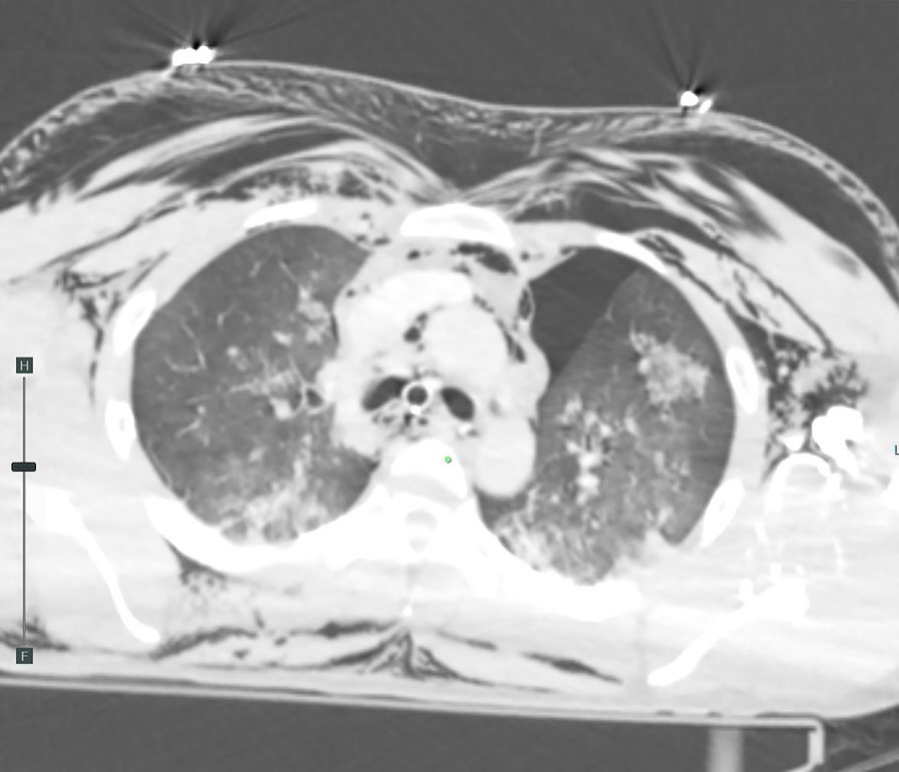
Computed tomography images showed the endotracheal tube inserted beyond the tracheal bifurcation
